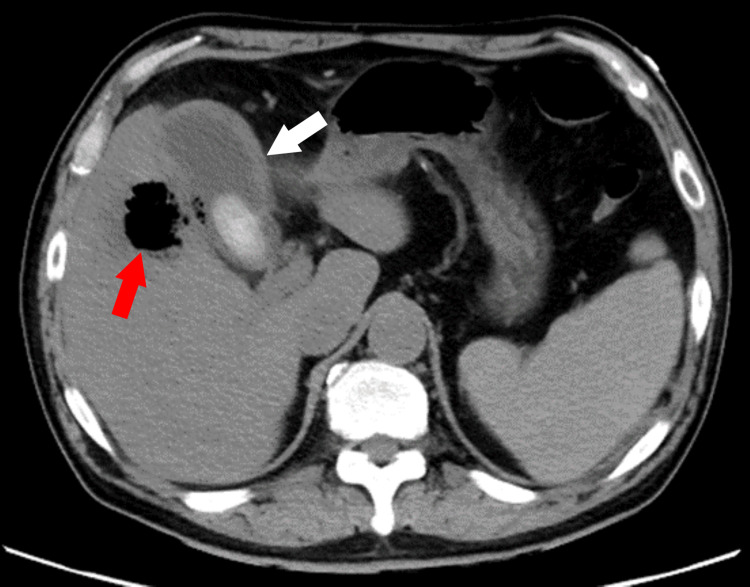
Abdominal CT scan demonstrating emphysematous cholecystitis (white arrow) and liver abscess (red arrow).
